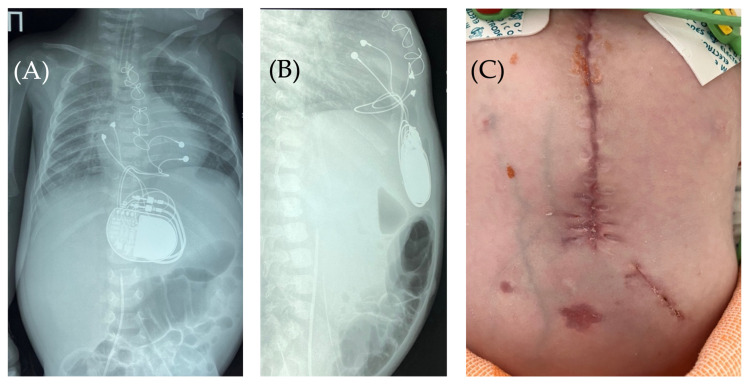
Chest X-ray after surgery debridement and closure of the wound. Chest X-ray (frontal (A) and lateral (B) view) shows no signs of pneumothorax, pneumopericardium, pneumomediastinum with minimal air at the pacemaker pocket site. (C) The healed sternotomy and the projection of the pacemaker pocket 12 days after revision and sanitation.
